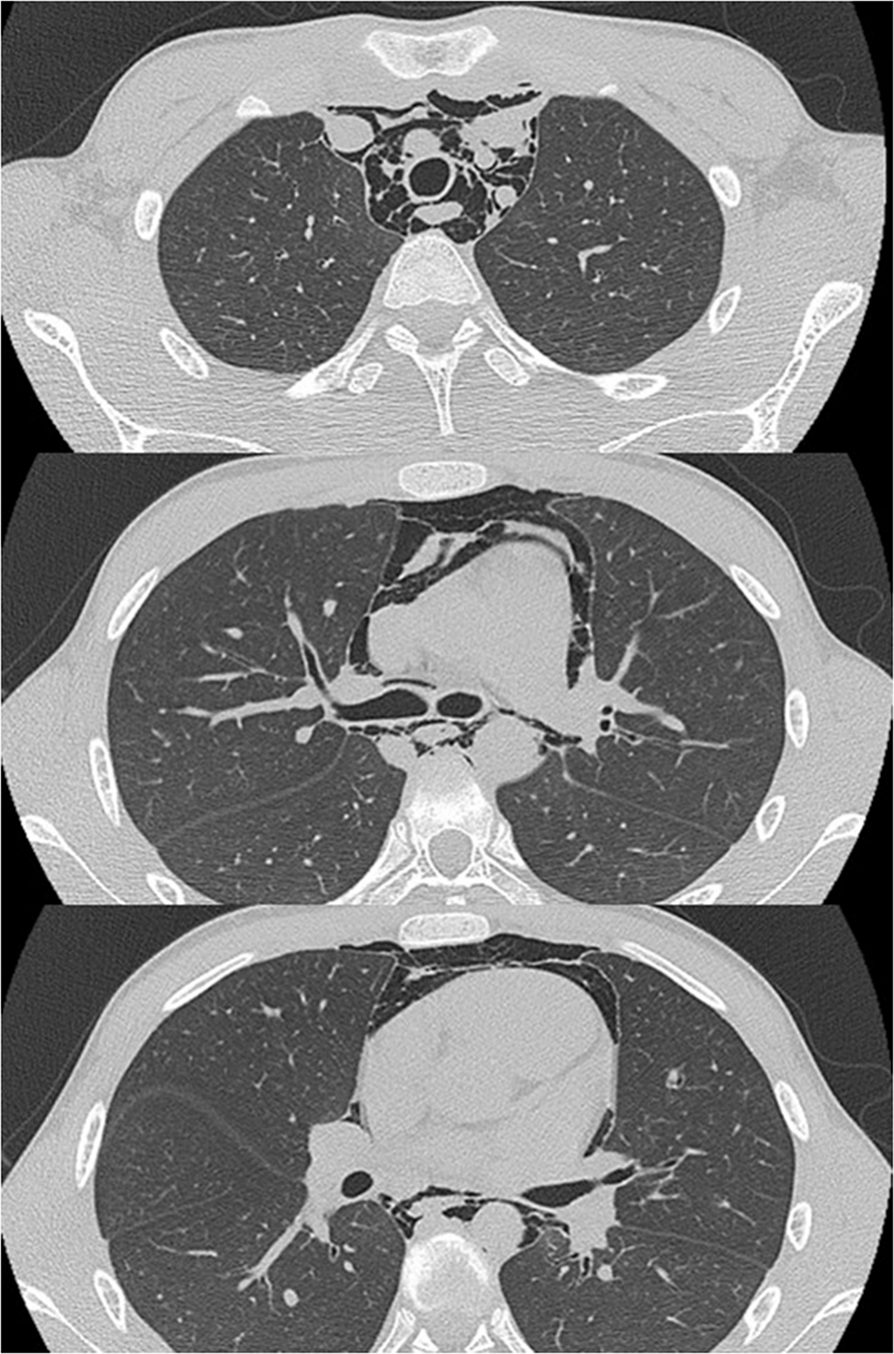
A computed tomography image showing massive pneumomediastinum surrounding the heart
